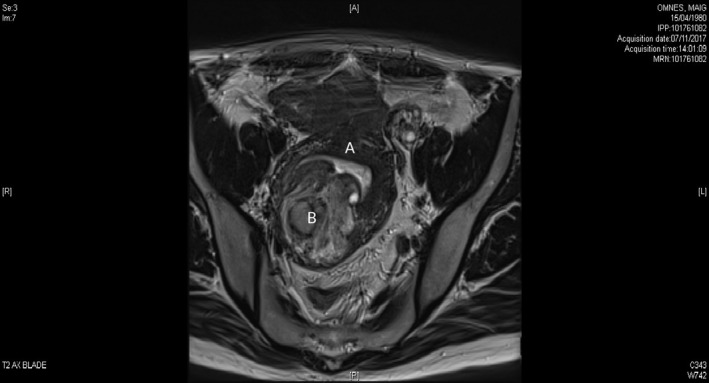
Cross section in MRI—T2‐weighted sequence: The uterine mass (B) deforms the uterine cavity, visible in the form of a parasol figure (A)
